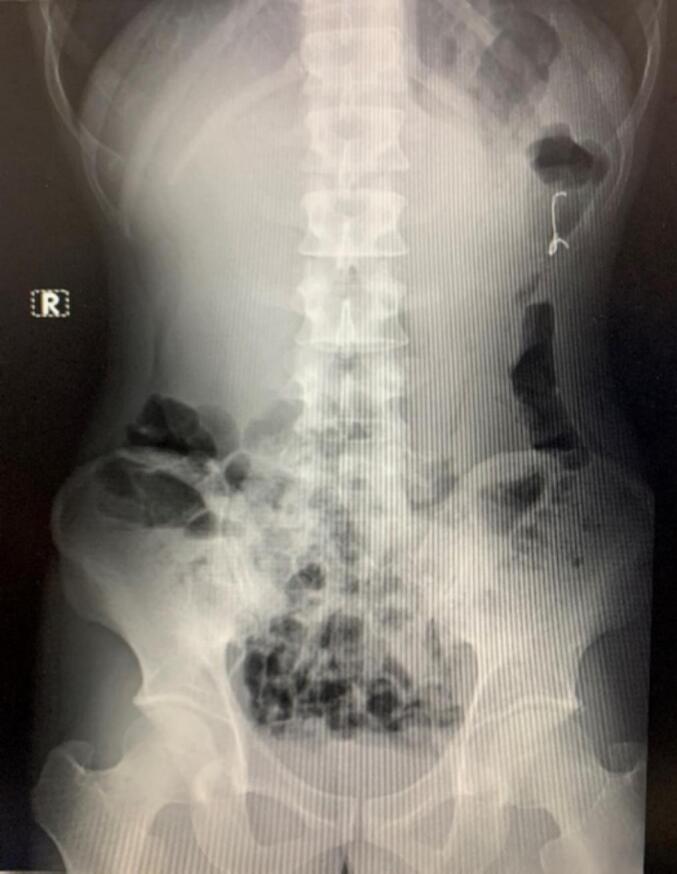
The abdominal X-ray upon admission showed a foreign body impacted in the descending colon.
